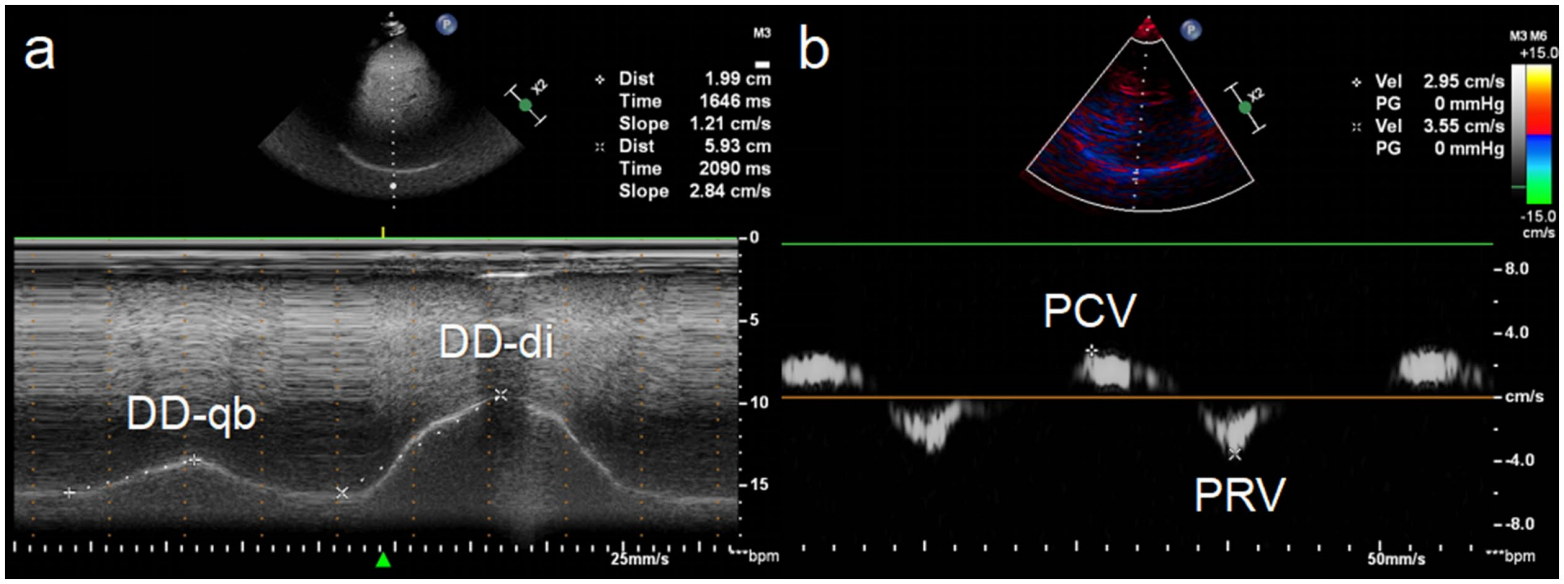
Ultrasonic measurement of diaphragm displacement (DD) and peak velocity. (a) The measured value of diaphragm displacement during quiet breathing (DD-qb) was 19.9 mm and during deep inspiration (DD-di) was 59.3 mm. (b) The measured value of peak contraction velocity (PCV) during inspiration was 2.95 cm/s and peak relaxation velocity (PRV) during expiration was 3.55 cm/s.
